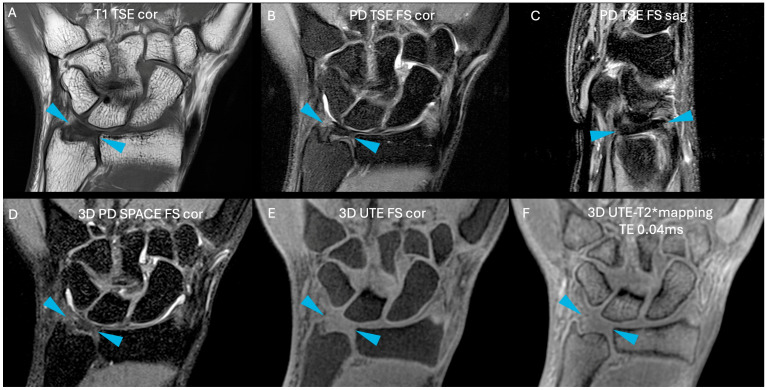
Example of all sequences acquired for the right side of a volunteer. (A) T1 TSE in coronal orientation; (B) PD TSE with fat suppression in coronal orientation; (C) PD TSE with fat suppression in sagittal orientation; (D) 3D PD SPACE with fat suppression in coronal orientation; (E) 3D UTE with fat suppression in coronal orientation; (F) and the first echo of the 3D UTE-T2* mapping sequence in coronal orientation (TE = 0.04 ms). Cortical bone appears hypointense on all sequences. Fluids appear hyperintense on T2- and PD-weighted images and hypointense on T1-weighted image. Fat suppression helps distinguish fluids from fat, as the latter is also hyperintense. Blue arrows indicate TFC.
